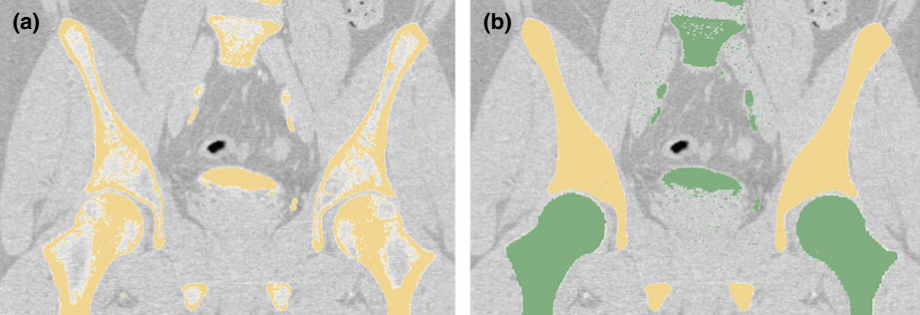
Human CT image, shown in the 3D slicer software package, used to create the pelvis model. (a) Initial threshold‐based segmentation (yellow). (b) Segmentation after the pelvis (yellow) has been separated from the remaining bones (green) and the cancellous bone sections filled in.
